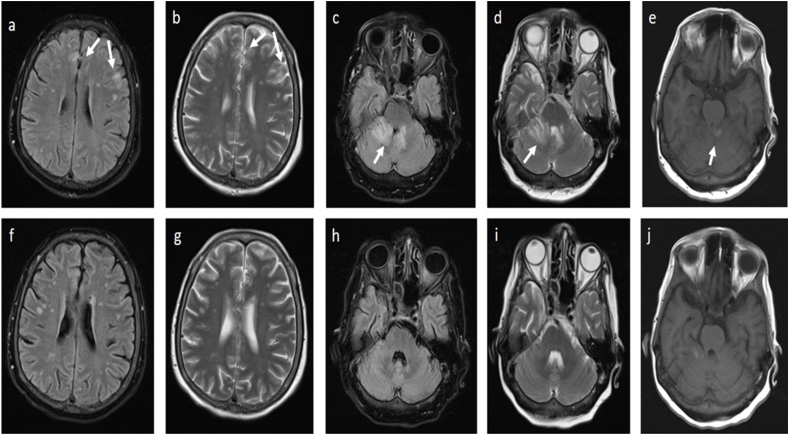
Post-COVID-19 vaccination magnetic resonance imaging (MRI) suggestive of encephalitis: Flair and T2 hypersignal changes in the cortical and subcortical areas of the frontoparietal lobes (a, b), cerebellar vermis, right cerebellar hemisphere and right middle cerebellar peduncle (c, d) without significant enhancement or restriction. Small focus of T1 hypersignality in the left side of the cerebellar hemisphere suggestive of hemorrhage (e). Post-treatment brain MRI (at 28 days after admission) showed subtle changes in MRI, in which signal changes disappeared (f, g, h, i, j).
